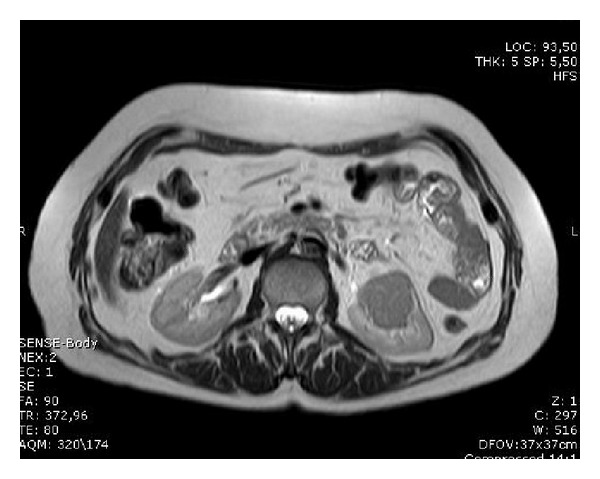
MRI abdominal showing hypernephroma of the left kidney.
